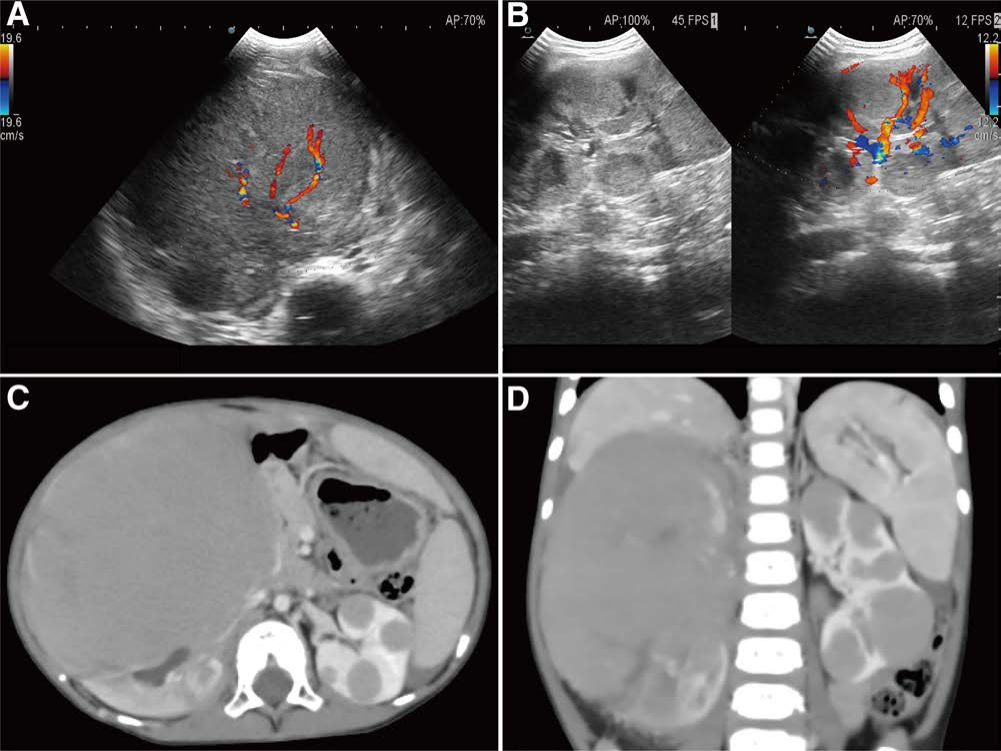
Ultrasonography revealed a solid heterogeneous hypoechoic mass in right abdomen (A); multiple homogeneous isoechoic nodules were seen in the contralateral kidney. (B) Enhanced CT showed the large solid low-density right mass infiltrated the entire right kidney, along with multi well defined nodules in left kidney, without enlarged lymph nodes or signs of metastasis (C and D).
